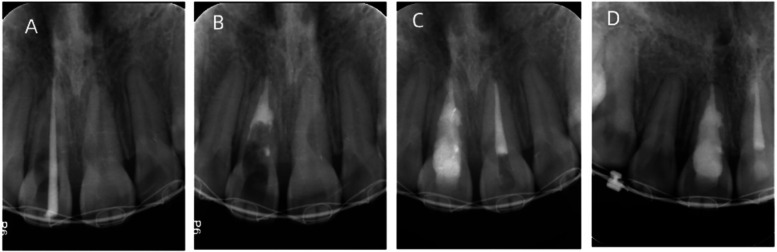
X-ray examination of the root canal treatment and a 3-year follow-up. (A) Working length determination. An x-ray shows that the working length is appropriate. (B) MTA is used for apical barrier and perforation repair. An x-ray shows that the apical 4 mm segment is tightly sealed with MTA as an apical barrier, and the lateral root perforation is repaired with MTA. (C) An x-ray taken right after root canal filling. The x-ray shows satisfactory root canal filling of the upper left central incisor and upper right central incisor. (D) At a 3-year follow-up, an x-ray reveals favorable prognosis in the upper left central incisor and upper right central incisor, with no periapical radiolucency observed.
